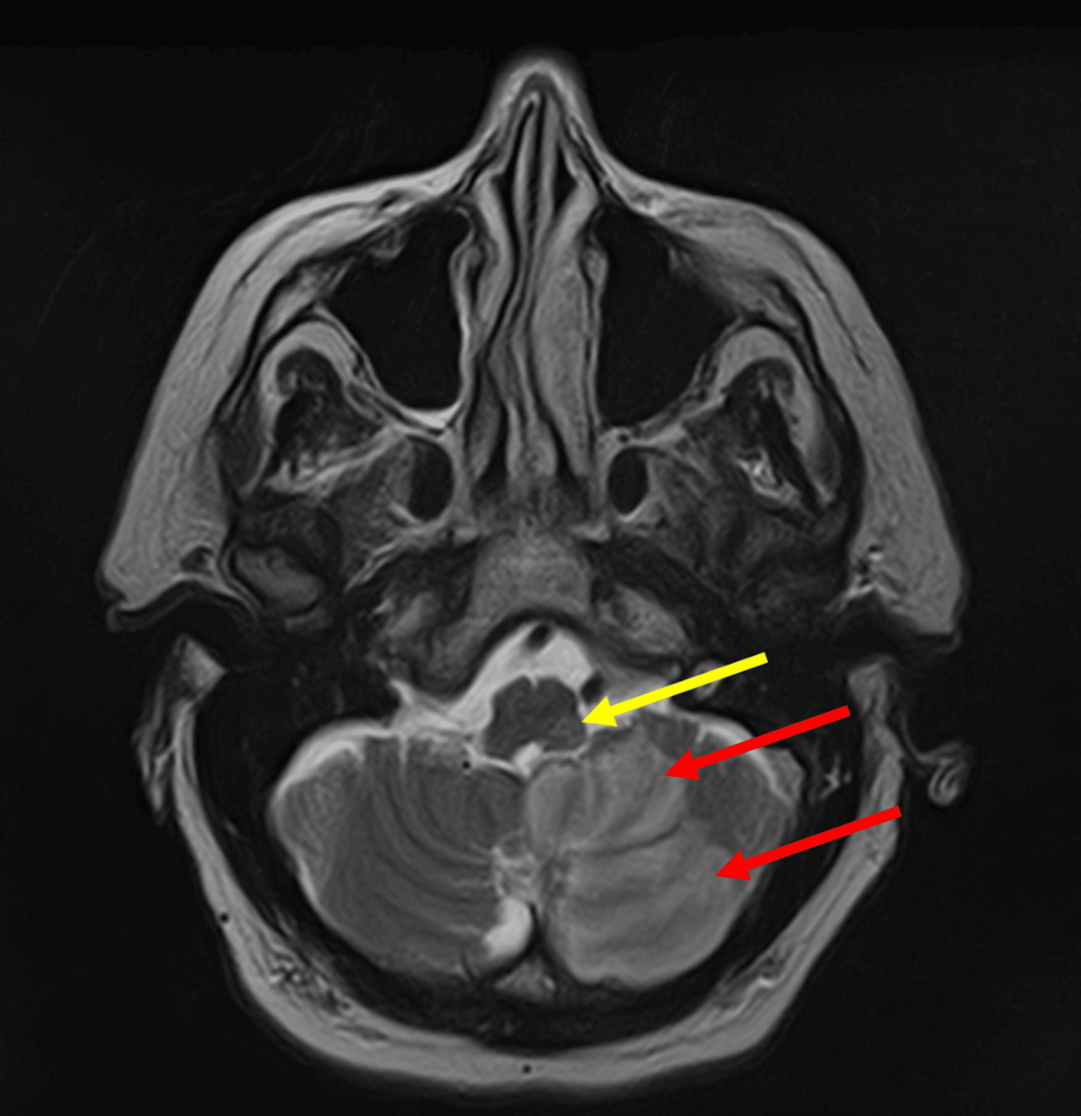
MRI of the brain taken on the second day of admission showing left PICA territory diffusion restriction (red arrows) with T2/FLAIR hyperintensity compatible with acute ischemic infarct, and diffusion restriction focus in the left dorsolateral aspect of the medulla oblongata (yellow arrow). No intracranial hemorrhage is seen. MRI: magnetic resonance imaging, PICA: posterior inferior cerebellar artery; FLAIR: fluid-attenuated inversion recovery
